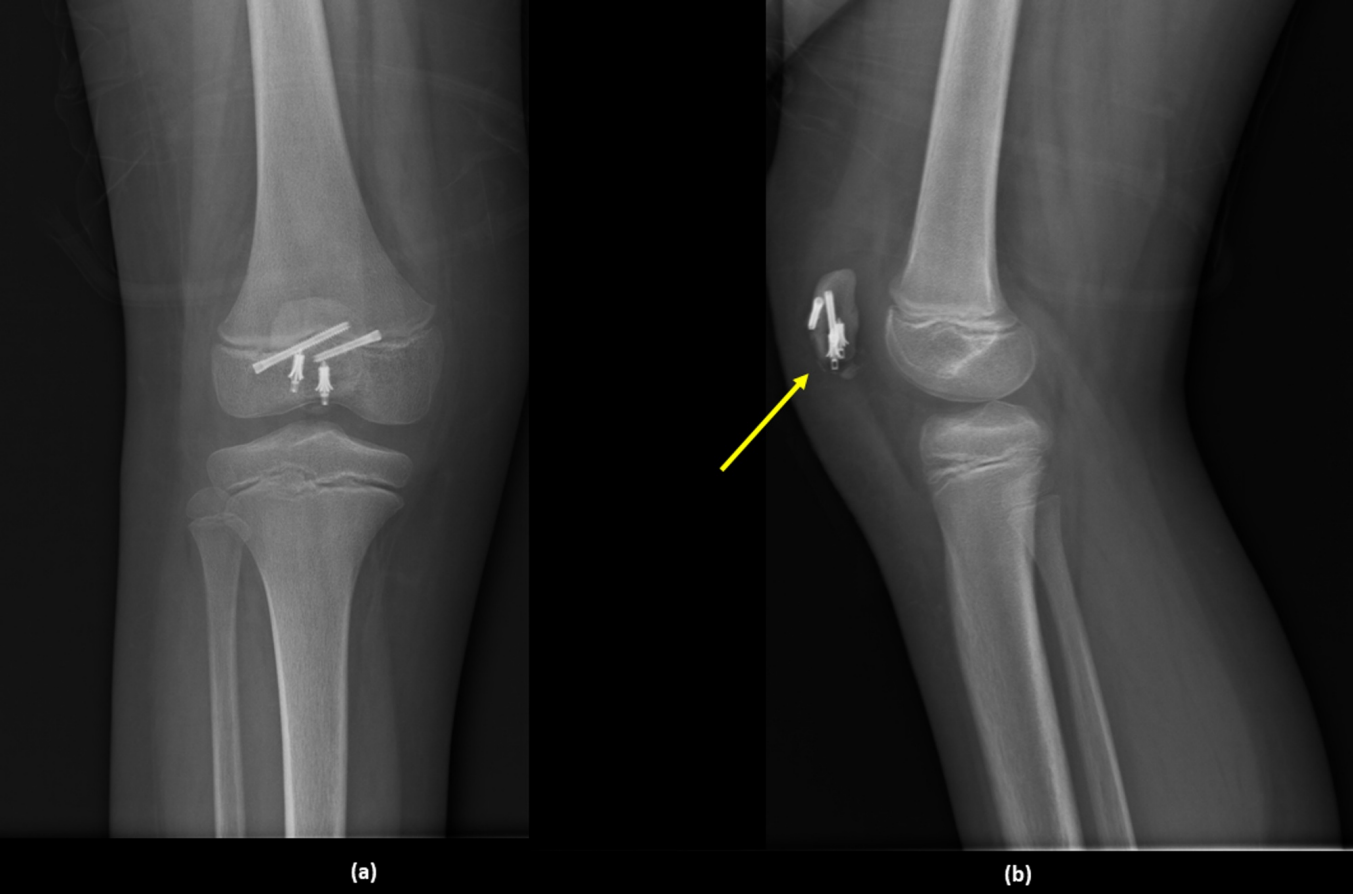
Radiographs at three-month follow-up: (a) anteroposterior view and (b) lateral view
